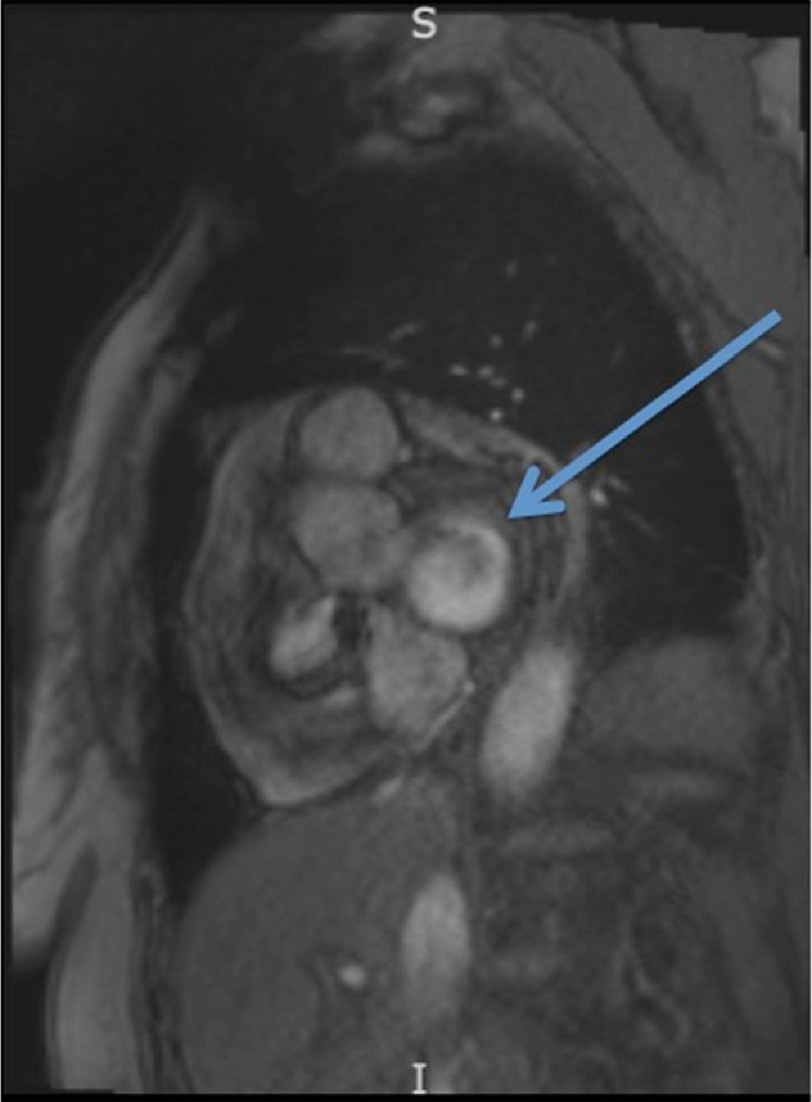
Sagittal view of the mass (indicated by the arrow) as seen on the patient’s cardiac MRI. Based on imaging findings, it was felt to be consistent with an atrioventricular node inclusion cyst.
